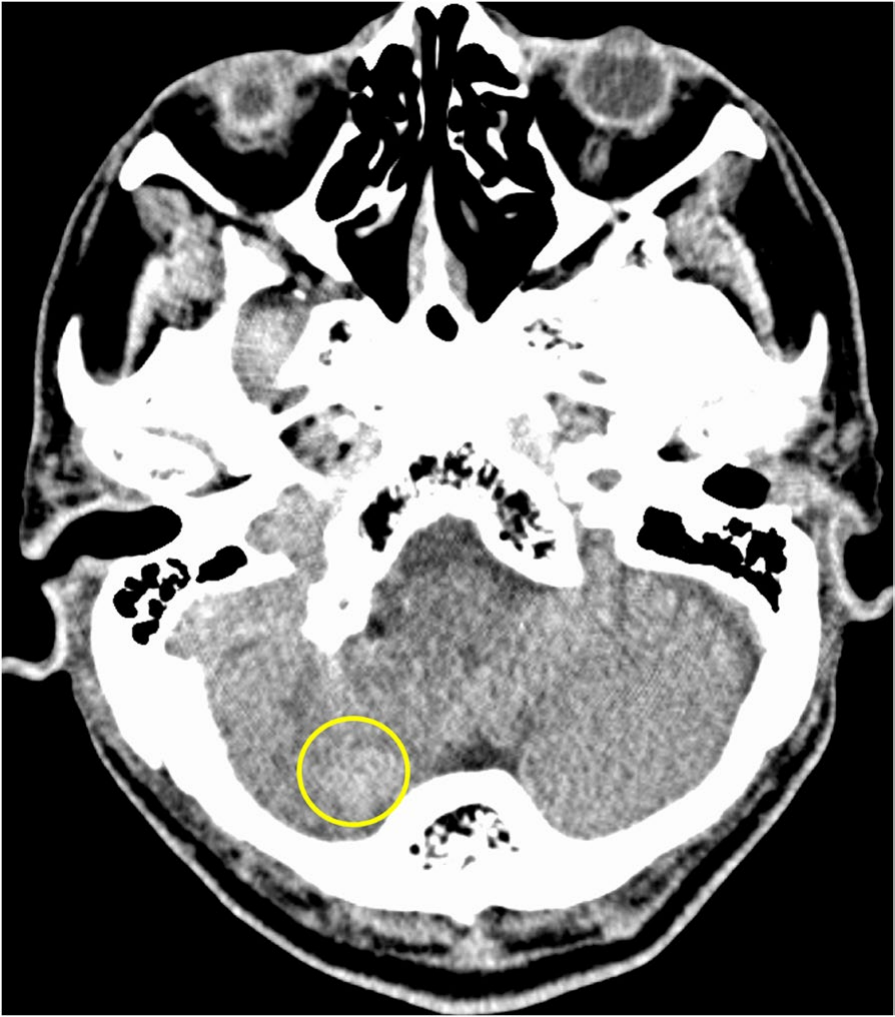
Brain computed tomography scan showing hemorrhagic infarction in the cerebellum on postoperative day 9 (yellow circle)
